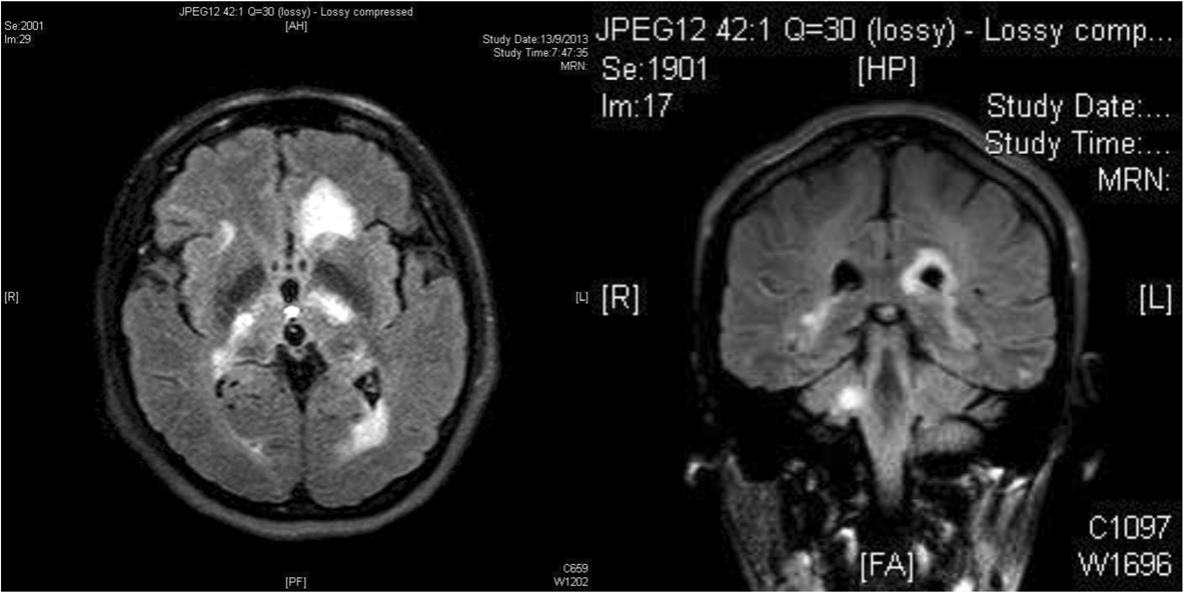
Magnetic resonance imaging of the brain with contrast performed on 13 September 2013. New periventricular and subcortical white matter of bilateral cerebral hemispheres, left-sided corpus callosum, left thalamus, left brainstem, and right cerebellar peduncle
